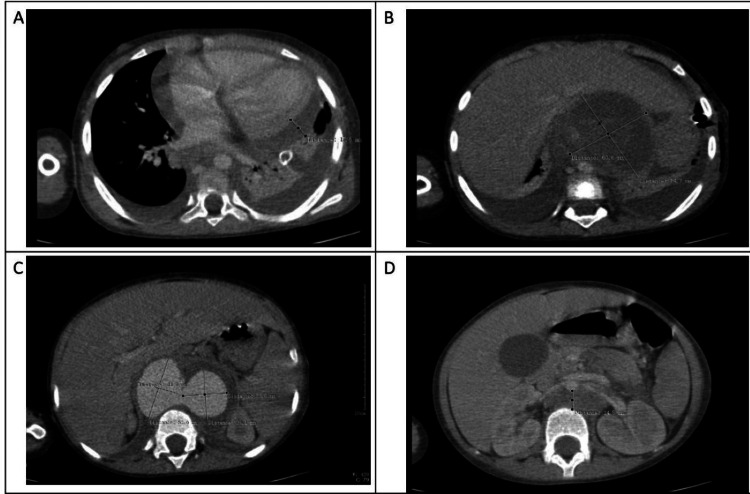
CT image showing (A) the pericardial effusion deepest pool 1.5 cm over the left ventricle (LV) free wall; (B) pseudoaneurysm alongside the abdominal aorta (6.8 x 7.1 cm) with air bubbles in it suggestive of an infected thrombus; (C) contrast pool on either side communicating with the abdominal aorta; and (D) aorta lifted off the spine by part of the thrombus (1.4 cm) with a normal caliber of the abdominal aorta at the mid-renal pool level.
